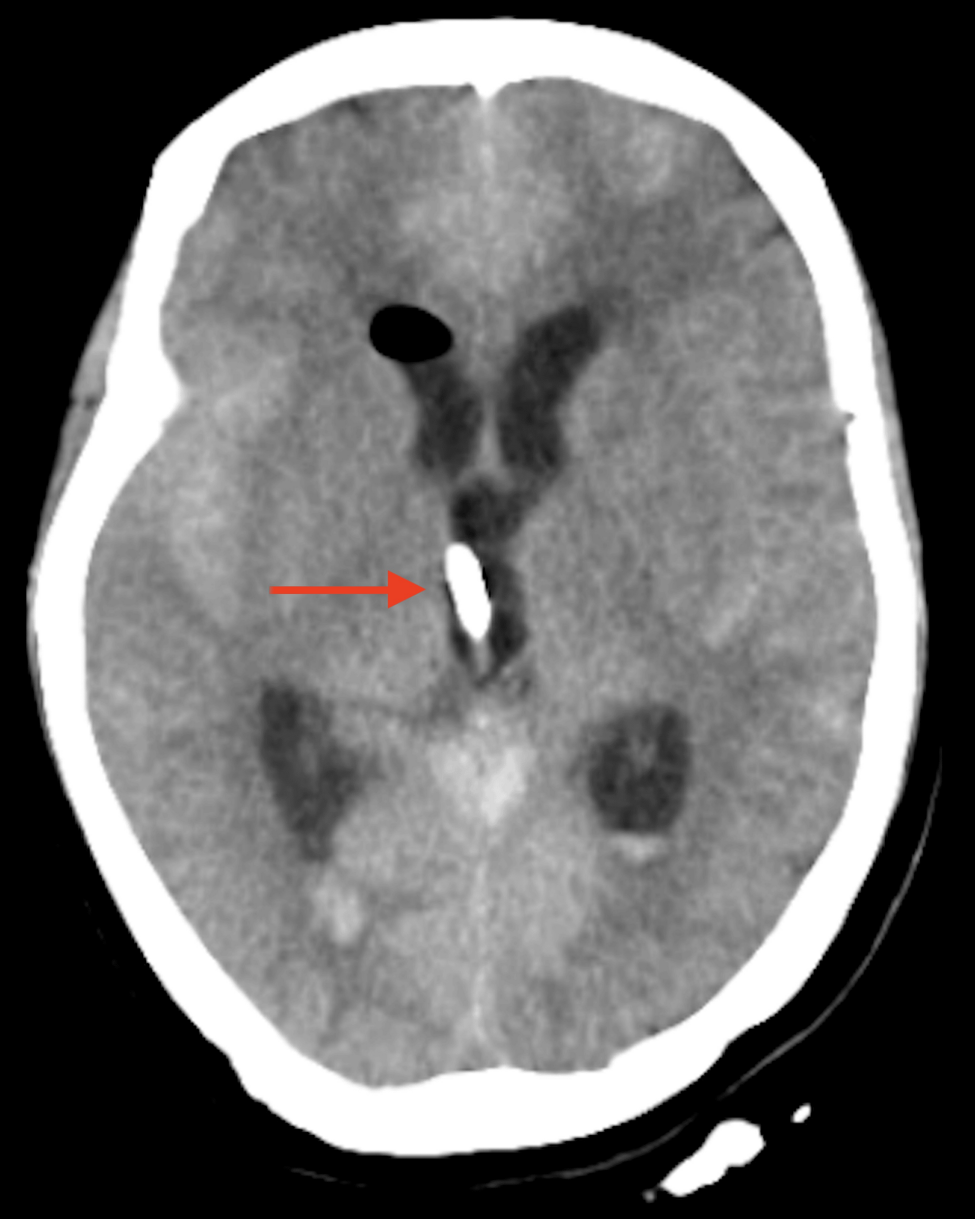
CT brain showing intraventricular hemorrhage in the third ventricle with red arrow along with subarachnoid hemorrhage.
